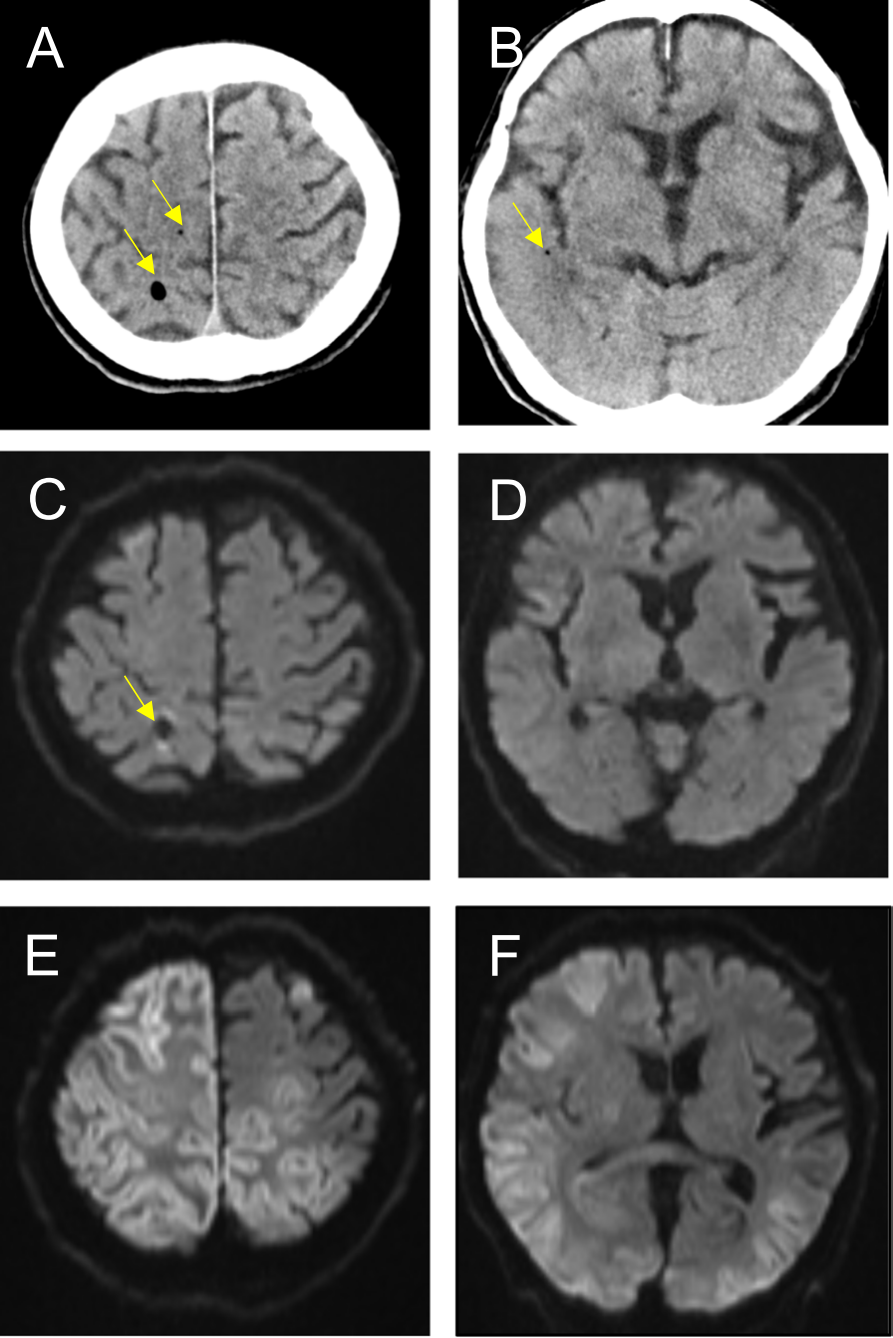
Evidence of cerebral gas embolism on neuroimaging. A, B A non-enhanced CT scan taken approximately 2 h after the start of partial nephrectomy. Air bubbles (arrows) were seen along the vessels of the cerebral surface. C, D MRI, including diffusion-weighted imaging sequences, performed approximately 3 h after the start of partial nephrectomy showed slight high intensity areas along the brain surface. E, F MRI performed on POD 7 showed extensive cerebral infarcts over the cerebral hemispheres bilaterally, consistent with the left conjugate eye deviation, epilepsy, and delayed emergence from anesthesia
